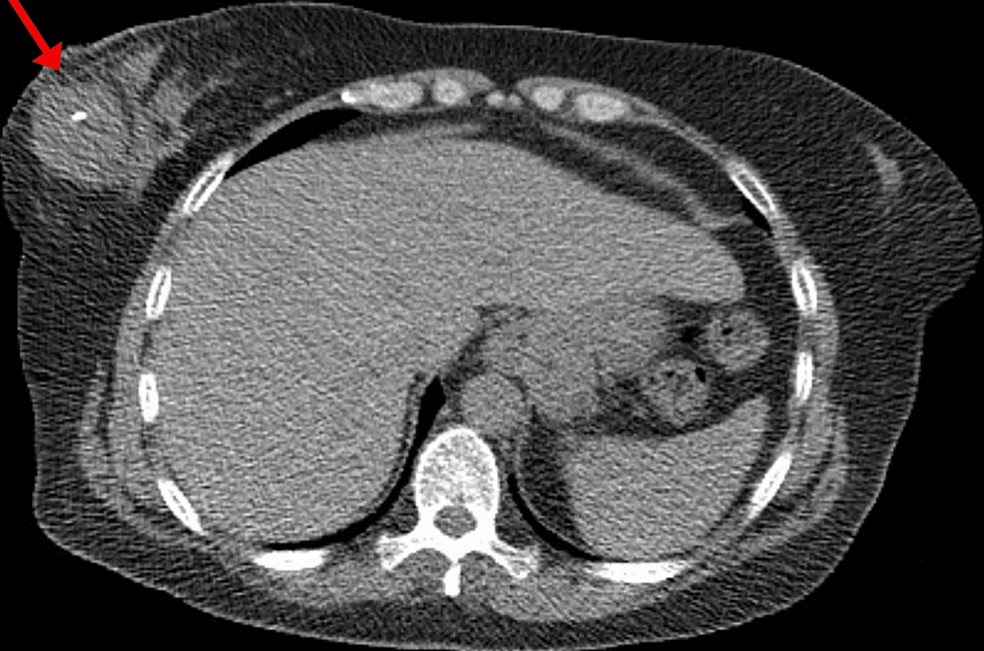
CT scan showing a 4.2-cm right breast mass (axial view)
